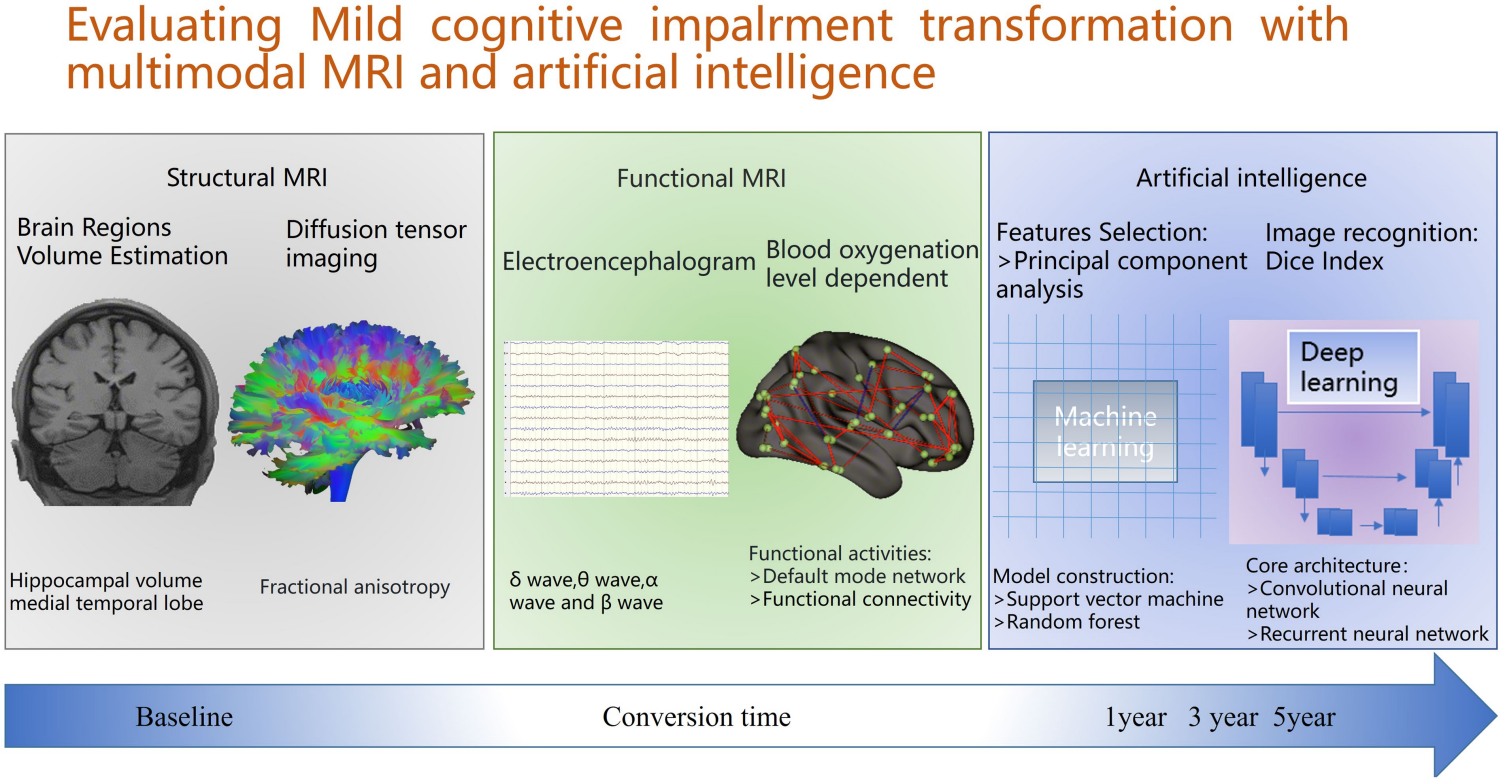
Overview of predicting conversion from mild cognitive impairment to Alzheimer’s disease: including structure MRI, functional MRI and artificial intelligence.
